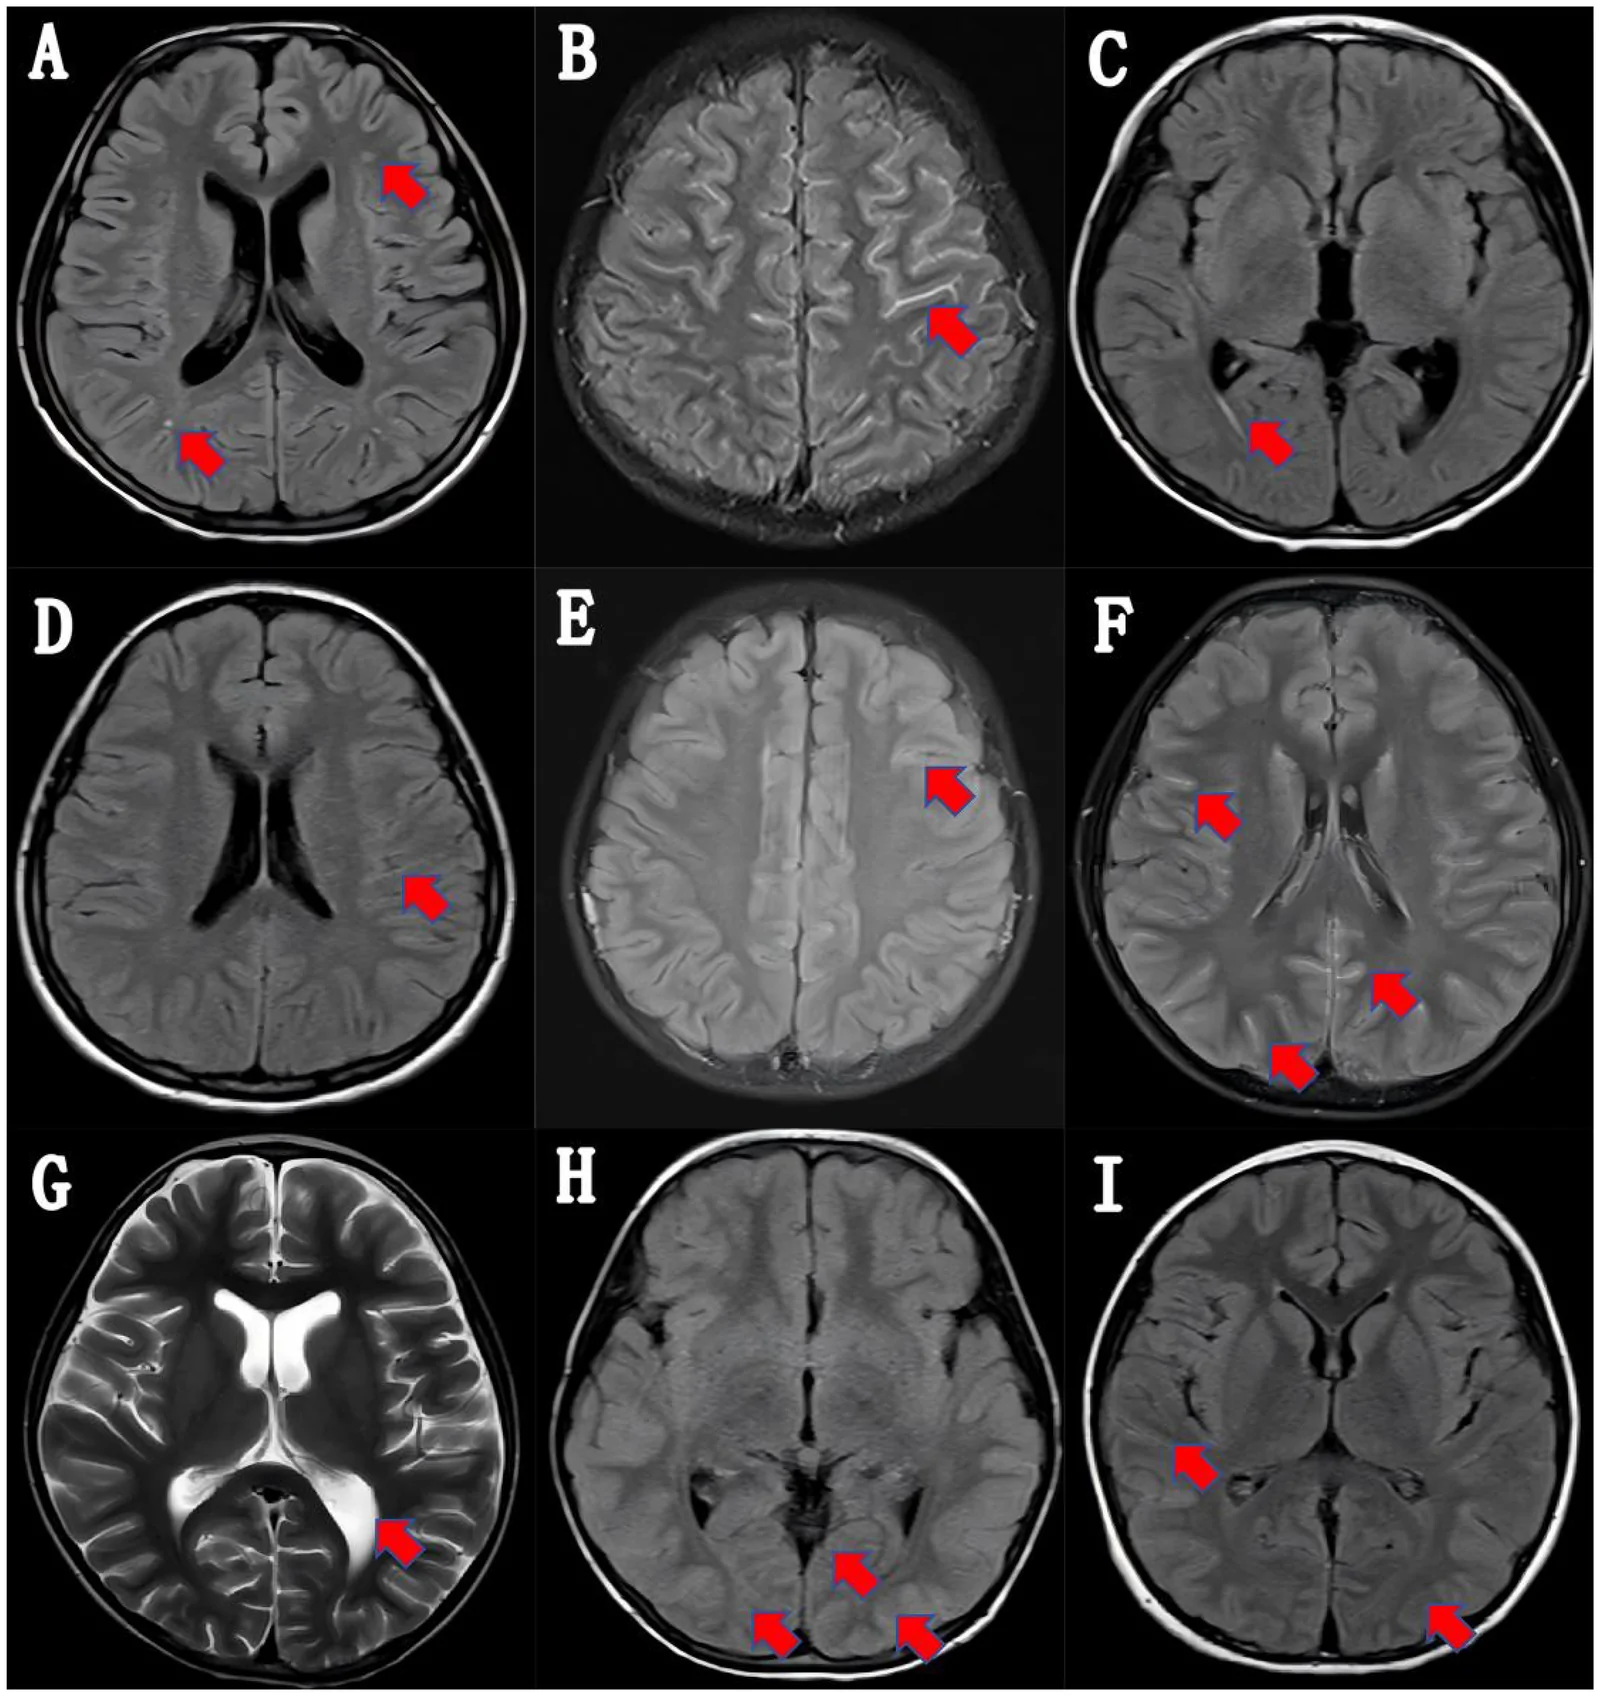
Brain magnetic resonance imaging (MRI) of patients with HNL-AM/ME. (A) Spotty or patchy distribution of abnormal signals in the frontal and occipital lobes; (B) Leptomeningeal enhancement sign; (C) Enlarged bilateral lateral ventricles, and hyperintensity in the right trigone on FLAIR; (D) Hyperintensity in the left frontoparietal sulci on FLAIR; (E) Widened cerebral sulci and mild focal LME; (F) Multifocal intracranial LME; (G) Widened cerebral sulci and mildly widened left occipital horn; (H) Gyral swelling of left parieto-occipital lobe, abnormal signals in left occipitoparietal and bilateral cingulate gyri, with linear hyperintense signals of sulci on FLAIR; (I) Widened cerebral sulci and hyperintensity in the right tempora sulci on FLAIR.
